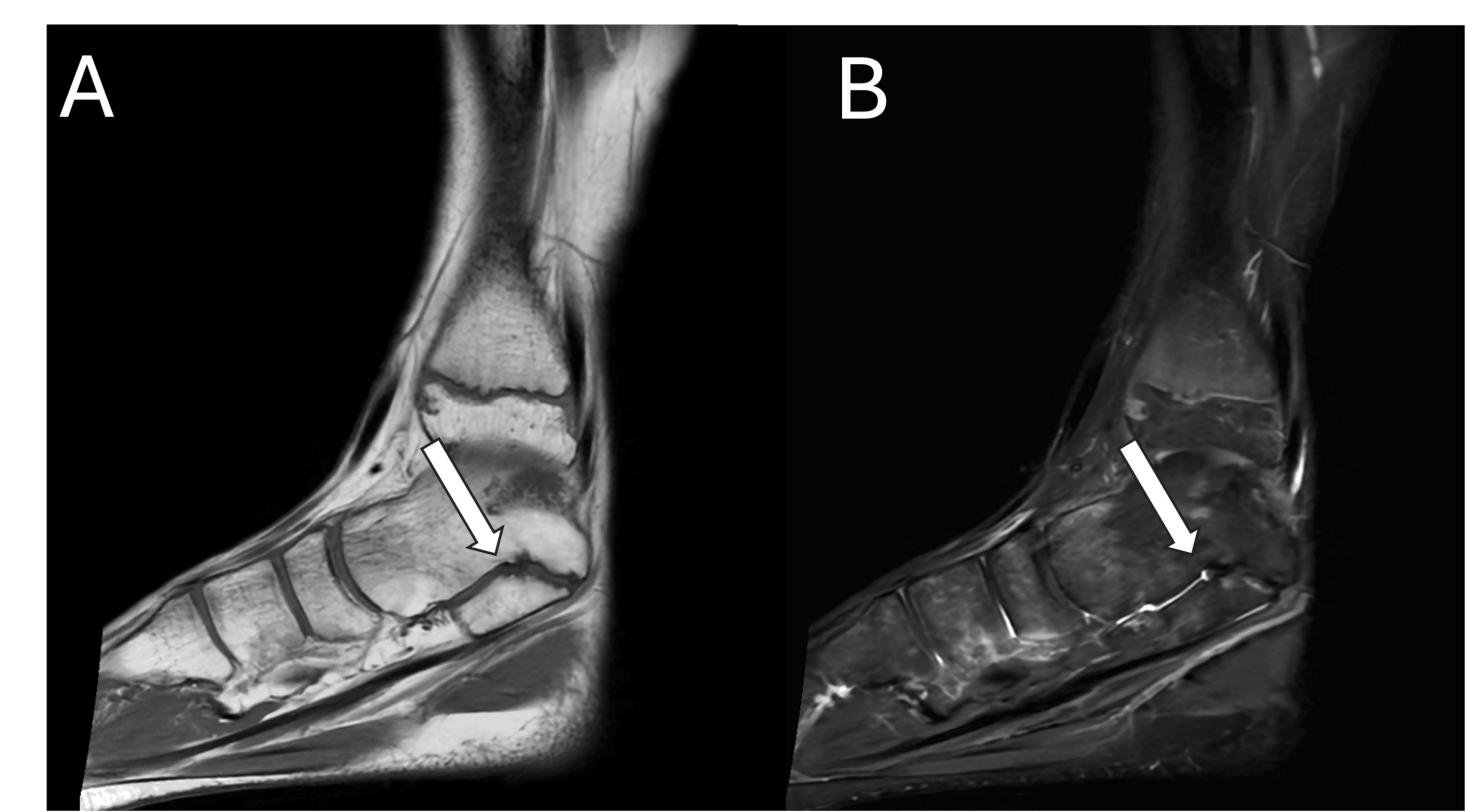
Sagittal (A) T1- and (B) T2-weighted slices of the left ankle without contrast, demonstrating a focal cortical irregularity and surrounding oedema at the medial margin of the TC joint, without continuity of the marrow signal, consistent with a non-osseous coalition shown with the white arrow.
